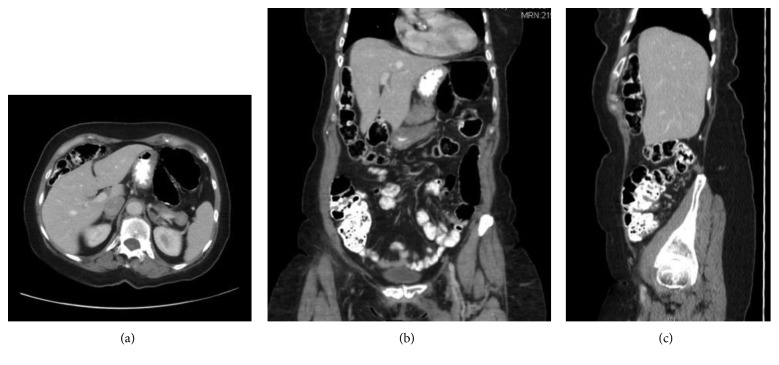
(a) Axial, (b) coronal, and (c) sagittal images from the patient's 2017 CT abdomen and pelvis demonstrating colon anterior to the liver.
